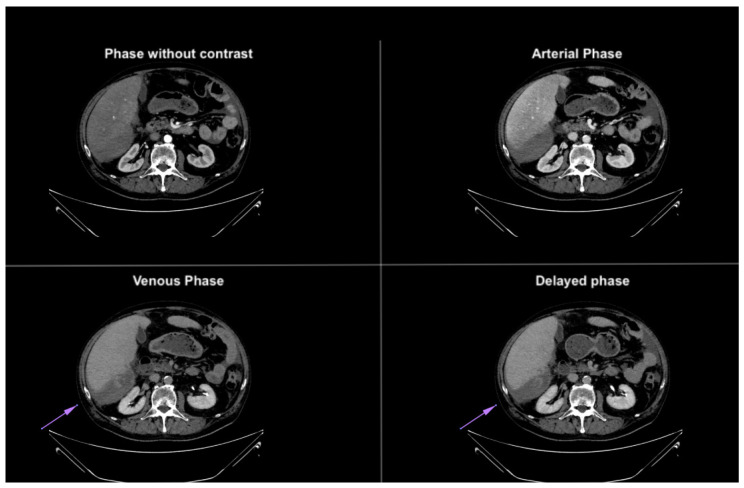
Axial CT images in different phases, highlighting extravasation of contrast medium within the subhepatic fluid collection. The extravasation becomes more prominent in the last phases, confirming the presence of active bleeding. The purple arrow demonstrates blood extravasation in the collection during the venous and delayed phases of the study, not visible in the baseline and arterial phases.
